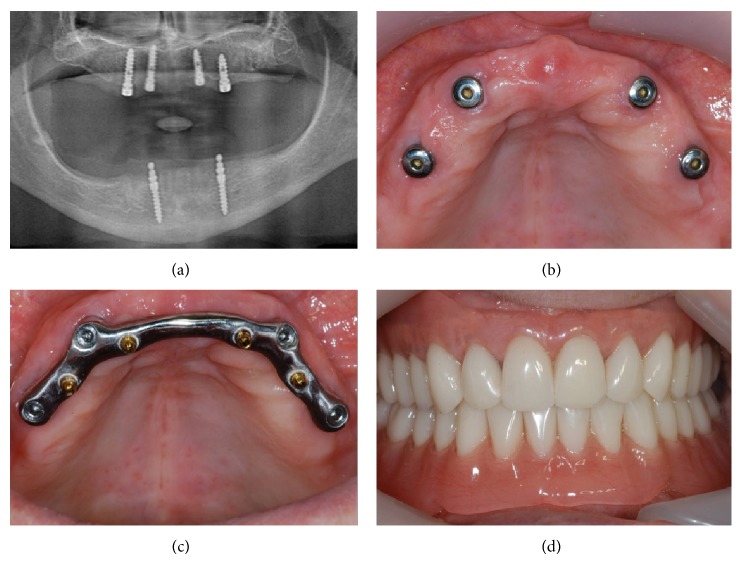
Surgical and prosthetic procedures: panoramic radiograph at implant placement (a); the implants after 4 months of undisturbed healing (b); the maxillary bar after application (c); clinical view of the maxillary overdenture (OD) at delivery (d).
